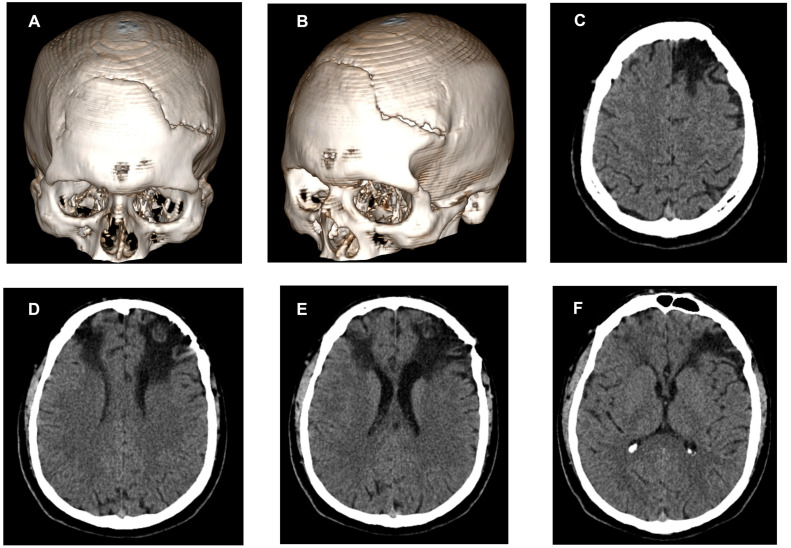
Computed tomography 3D bone reconstruction (A,B) and axial computed tomography of the brain (C–F). A post-traumatic bone defect of the skull can be seen in (A–F) shows changes of encephalomalacia in the frontal lobes’ parenchyma, affecting both white and grey matter, more extended on the left side, with enlargement of the anterior ventricular horn (aspect of acquired porencephalic lesions).
